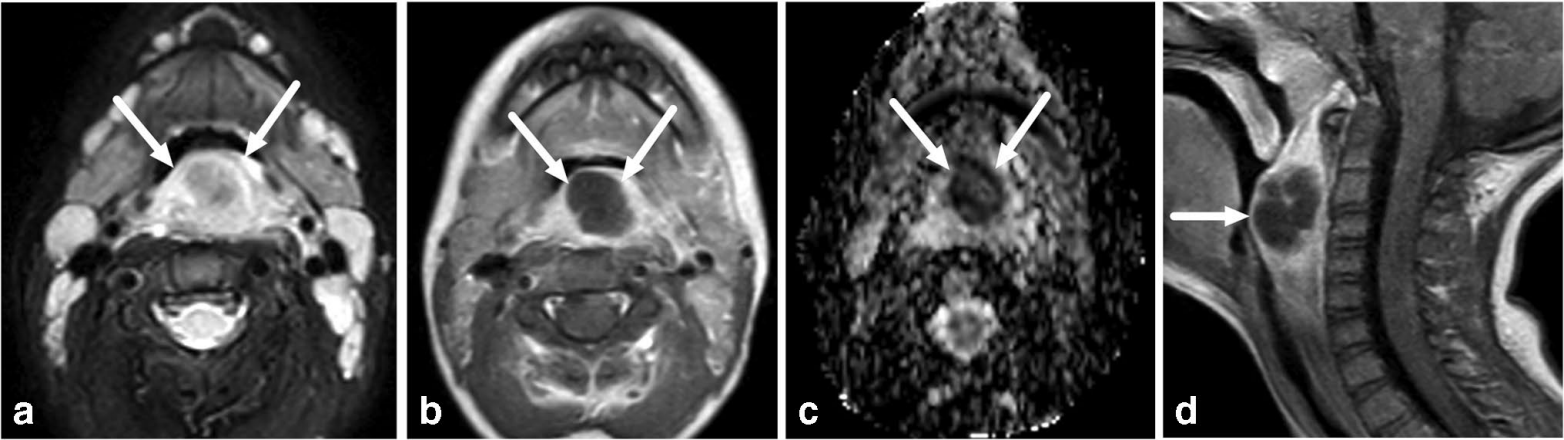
True retropharyngeal abscesses in a 3-year-old boy with throat pain and swelling, who had a bulging abscess (*arrows*) in the median retropharyngeal space. a Axial T2-weighted with fat saturation. b Axial T1-weighted post-contrast. c Axial apparent diffusion coefficient. d Sagittal T1-weighted post-contrast
